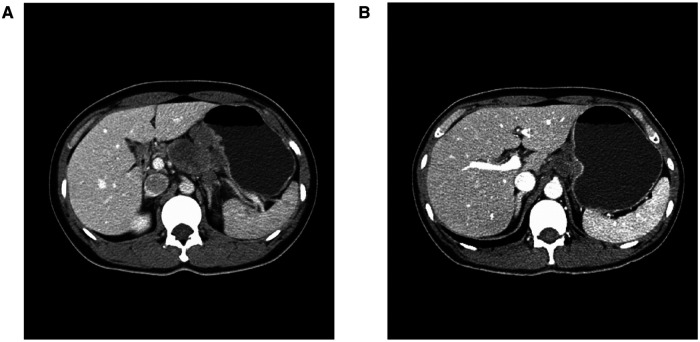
Abdominal CT imaging (A) Before chemo-immunotherapy; (B) After 3 courses of chemo-immunotherapy.
